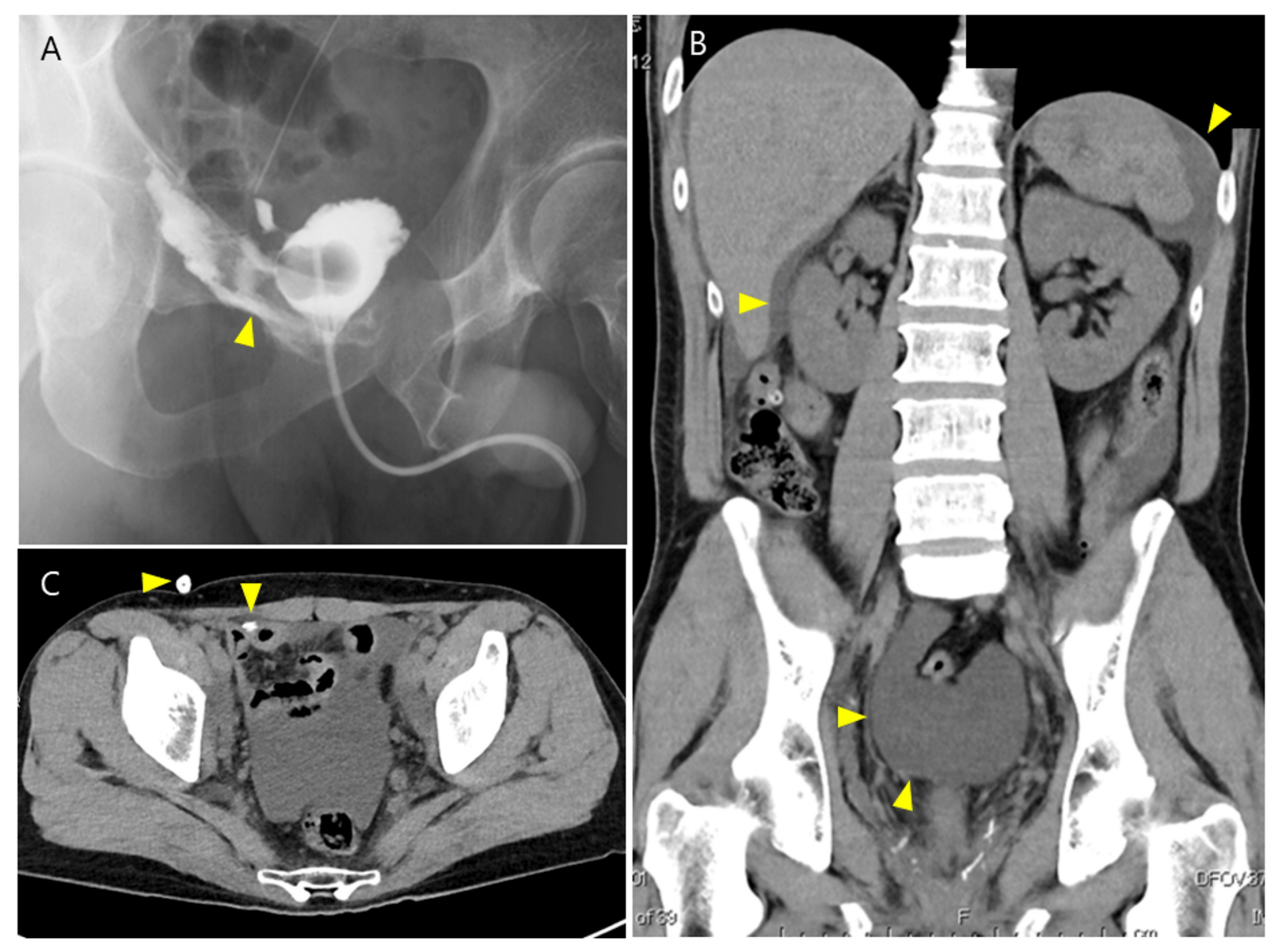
(A) Cystography on POD 8 demonstrating significant enlargement of the anastomotic leakage. The arrowhead indicates contrast leakage. (B) Abdominal CT scan on POD 9 showing fluid accumulation around the bladder, liver, and spleen. (C) Ventral migration of the drain, impairing urinary drainage. The arrowhead indicates the position of the drain. POD, postoperative day
